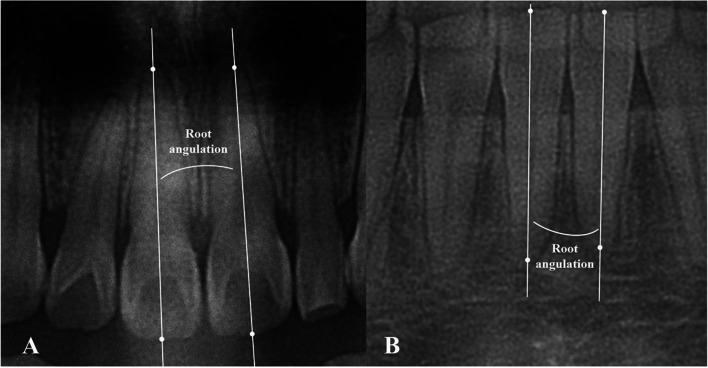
Measurement of root angulation on panoramic radiographs after treatment. A Root angulation of maxillary central incisors. B Root angulation of mandibular central incisors
